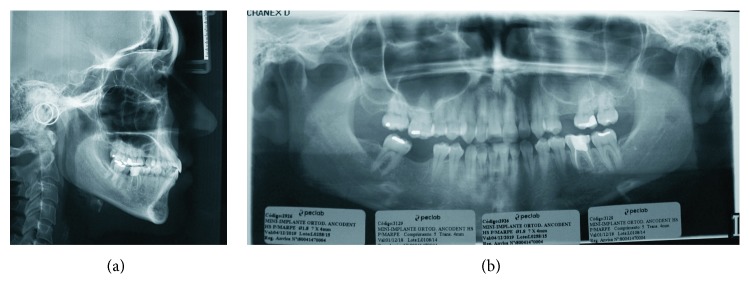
Lateral X-ray (a). Panoramic X-ray (b).
